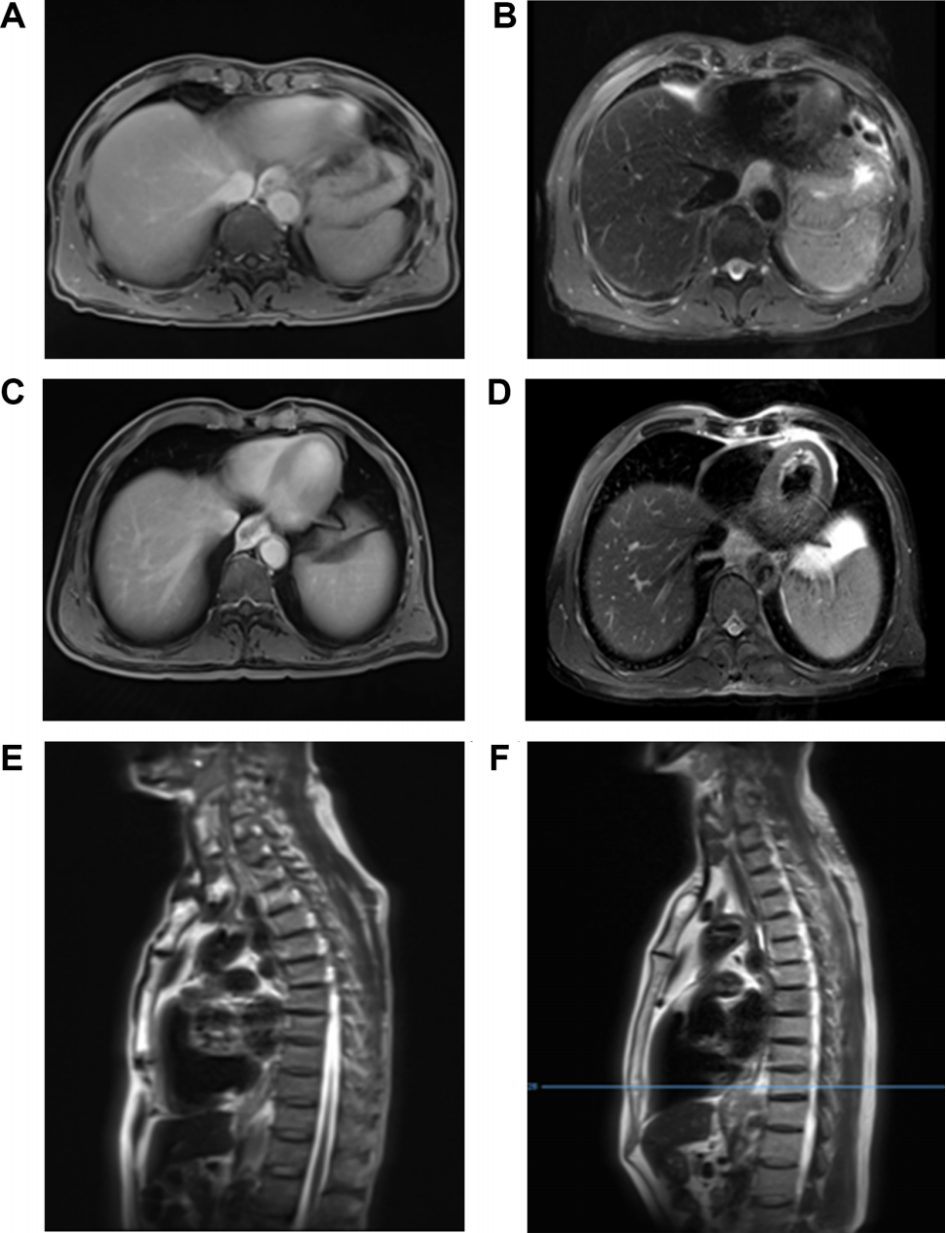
Magnetic resonance images of 2 samples. T2-weighted (A), fat-suppressed T2-weighted (B), MRIs (E) of stage IIB; T2-weighted (C), fat-suppressed T2-weighted (D), MRIs (F) of stage IIIB. MRI indicates magnetic resonance imaging.
